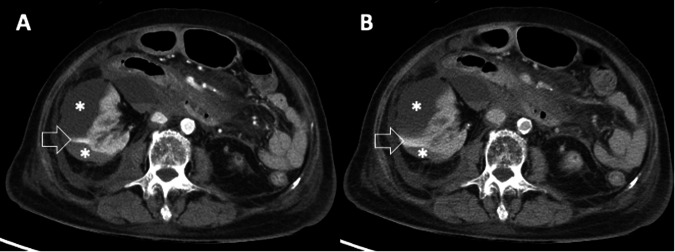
CT images (A; arterial phase, B; portovenous phase) exemplifying path analysis alignment in a deceased patient during admission. An 80-year-old woman with end-stage renal disease on hemodialysis presented with spontaneous right perinephric hematoma, having prolonged serum partial thromboplastin time (180 s). The CT revealed active vascular contrast extravasation, featuring a minimum length of 11 mm on portovenous phase. She received 4 units of packed red cells within the initial 24 h, was admitted to the intensive care unit for nonsurgical care, and subsequently succumbed after 90 days of admission
